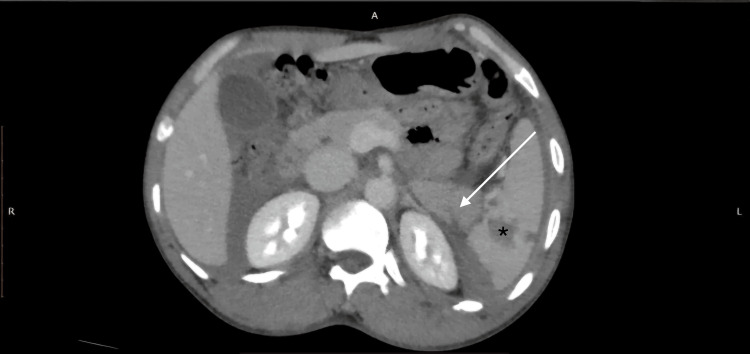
AAST grade I pancreatic injury with superficial laceration and grade III splenic injury with subcapsular hematoma on CECT (PV protocol). A 35-year-old male with a history of alcohol intoxication under unknown circumstances underwent CECT of the abdomen using the PV protocol. The axial CECT image shows a small superficial pancreatic laceration (white arrow) without ductal injury. Additionally, the spleen exhibits an intraparenchymal laceration (black star) extending to the splenic hilum, with a subcapsular hematoma but no active contrast extravasation. Moderate hemoperitoneum was also noted. Findings were consistent with the AAST grade I pancreatic injury and grade III splenic injury. AAST: American Association for the Surgery of Trauma; CECT: contrast-enhanced computed tomography; PV: portal venous.
